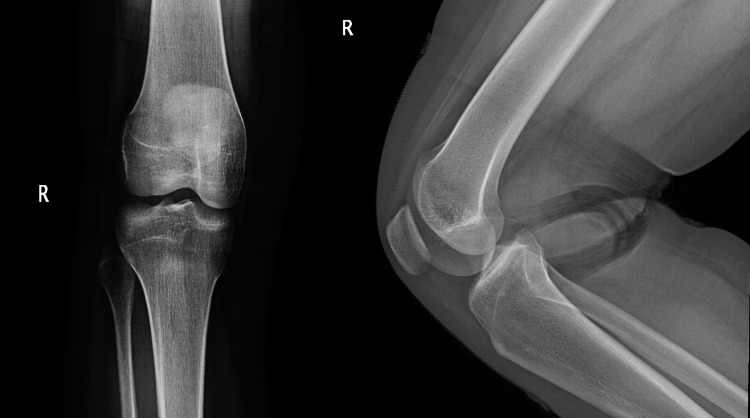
Anteroposterior and lateral right knee radiographs showed no bony abnormality.
